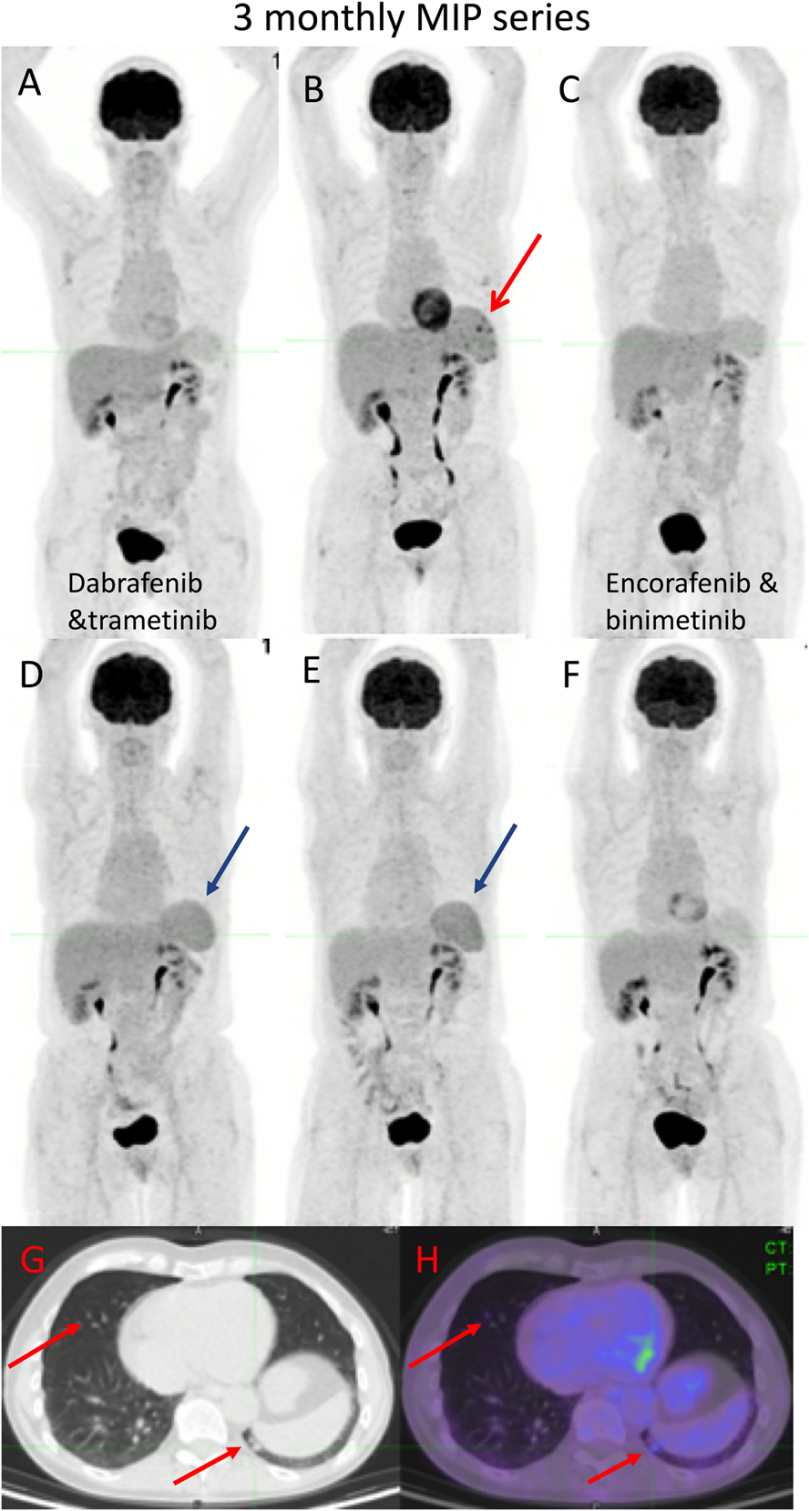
68-year-old female with primary melanoma in the upper back (1 mm Breslow thickness, no ulceration, mitotic rate < one, no peri-neural or lymphovascular invasion) with negative sentinel node biopsy at diagnosis (5 years ago) who developed self-detected right axillary metastasis one year later (images not provided). Axillary nodal dissection demonstrated 1/9 lymph nodes involved with 8 mm deposit and 0.3 mm extra-nodal extension (stage IIIB) BRAF V600K. Started on ICI (Ipilimumab and Nivolumab) afterwards and developed multifocal acquired demyelinating sensory and motor neuropathy treated with steroids, plasmapheresis and rituximab. Surveillance ^18^F-FDG PET/CT (A) showed new small pulmonary nodules (not evident on MIP),red arrows axial fused image(G and H); which were confirmed to be metastatic melanoma on wedge resection. MRI brain also showed small new cerebral metastases (stage IV-M1b). Treatment subsequently changed to Dabrafenib and Trametinib, which was poorly tolerated with recurrent episodes of fever, myalgia and fatigue. Surveillance ^18^F-FDG PET/CT scans were performed 3 monthly afterwards; the 1st follow-up ^18^F-FDG PET/CT showed an enlarged spleen with multiple foci of moderately increased FDG uptake (red arrow - B). The treatment regimen changed to 4 days on and 3 days off; however, this was also poorly tolerated and the patient was switched to Encorafenib and binimetinib with subsequent scans showing a mild interval splenic enlargement and reversal of the spleen to liver activity (blue arrows – D,E). The patient remained in CMR on subsequent scan and reversal of spleen to hepatic activity normalised
